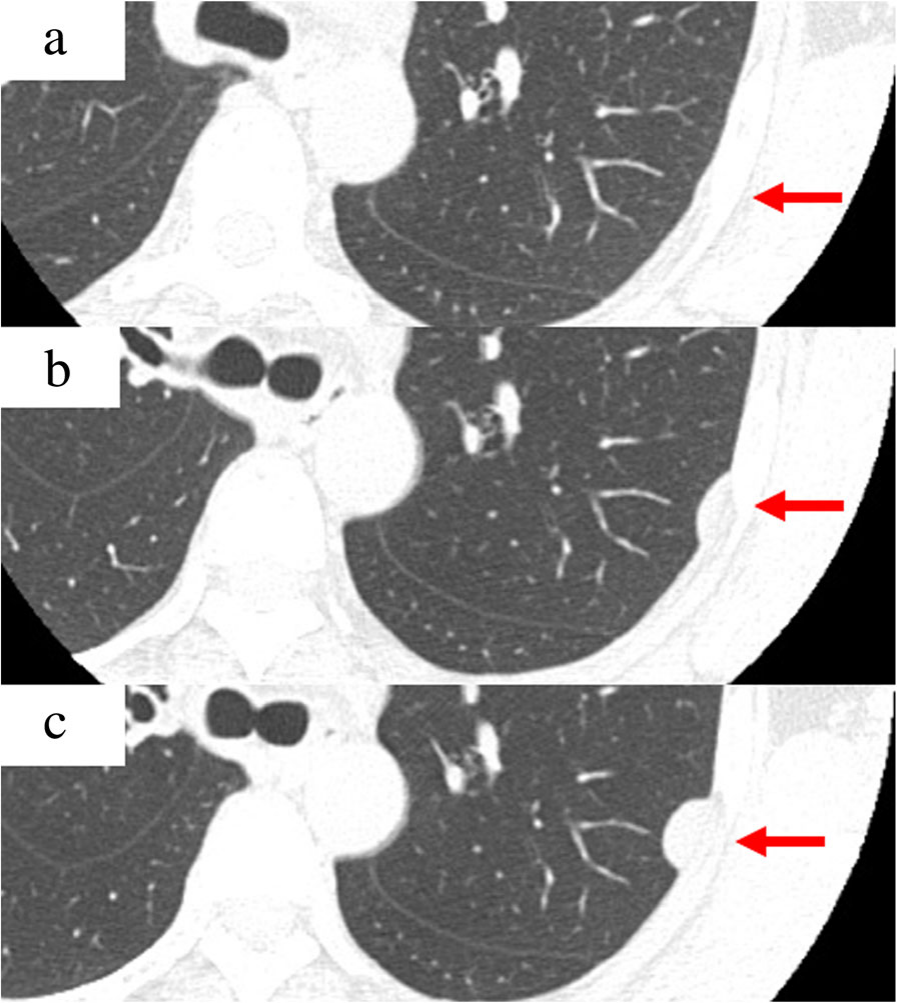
Computed tomography demonstrated a gradually increasing low-density mass (*arrows*) measuring 2.0 × 1.8 cm in diameter. There was no mass region at 6 months after the wedge resection of the lower lobe of the left lung (a). A flat region appeared 1 year after the wedge resection of the lower lobe of the left lung (b) with increasing size up to 2.0 × 1.8 cm at 2 months before the present surgery (c)
